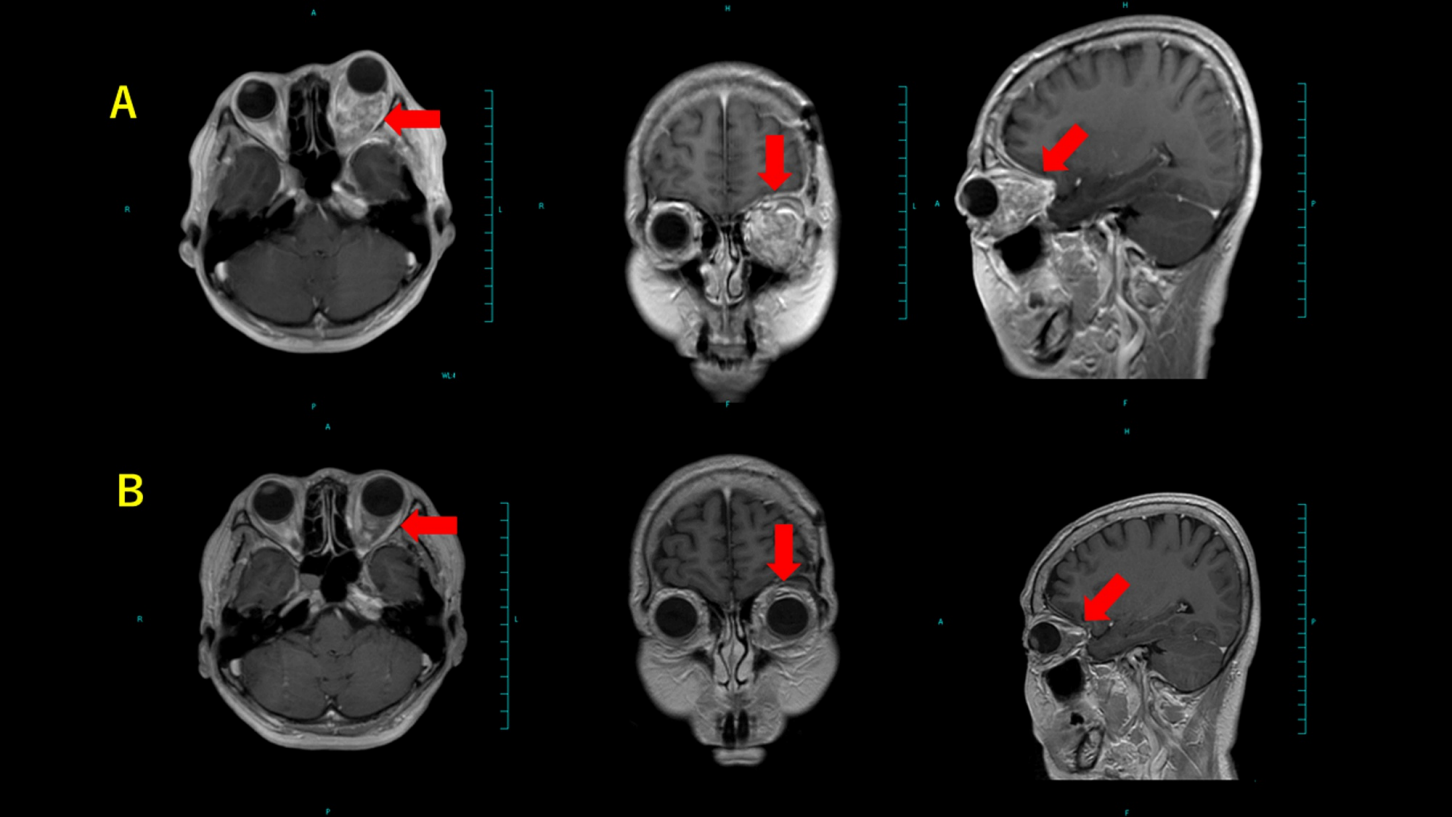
Brain MRI of case 1 (A) Before treatment and (B) 84 months after treatment. Red arrows denote tumor.
